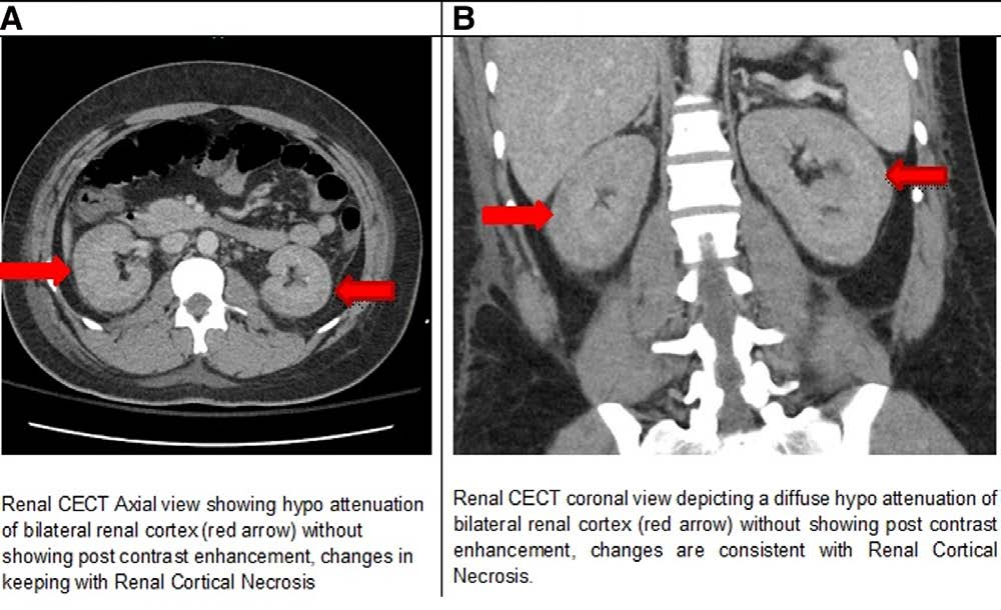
(A) Kidney contrast enhanced CT scan: axial section depicting renal cortical necrosis. (B) Coronal section depicting renal cortical necrosis.
